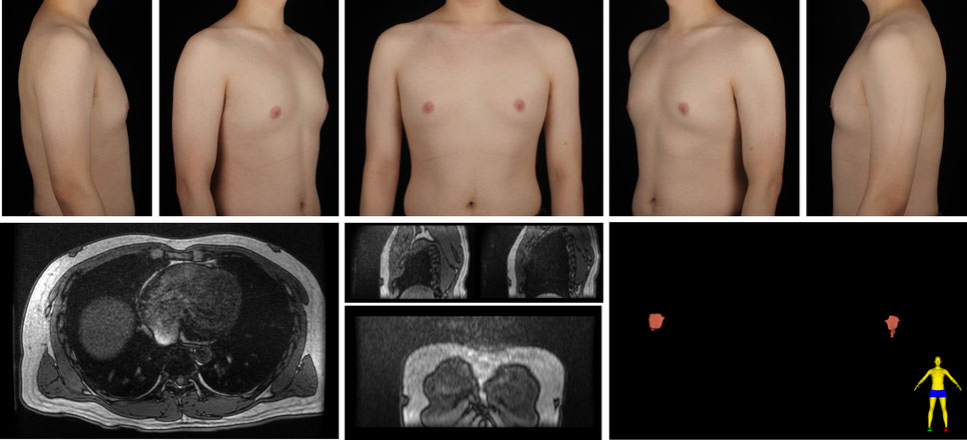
Type 1C gynecomastia. Preoperative chest appearance (upper) and breast MR images (lower) of a 30-year-old man with type 1C gynecomastia
